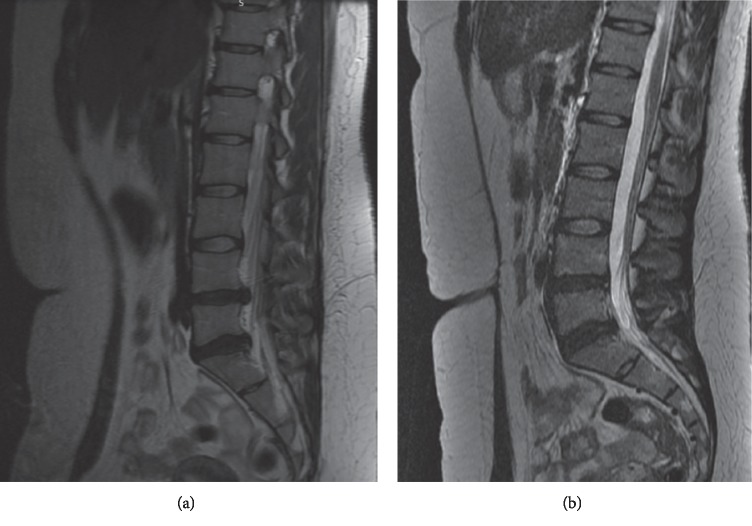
MRI. Date of examination: (a) 4^th^ December 2018 and (b) 20^th^ September 2019.
